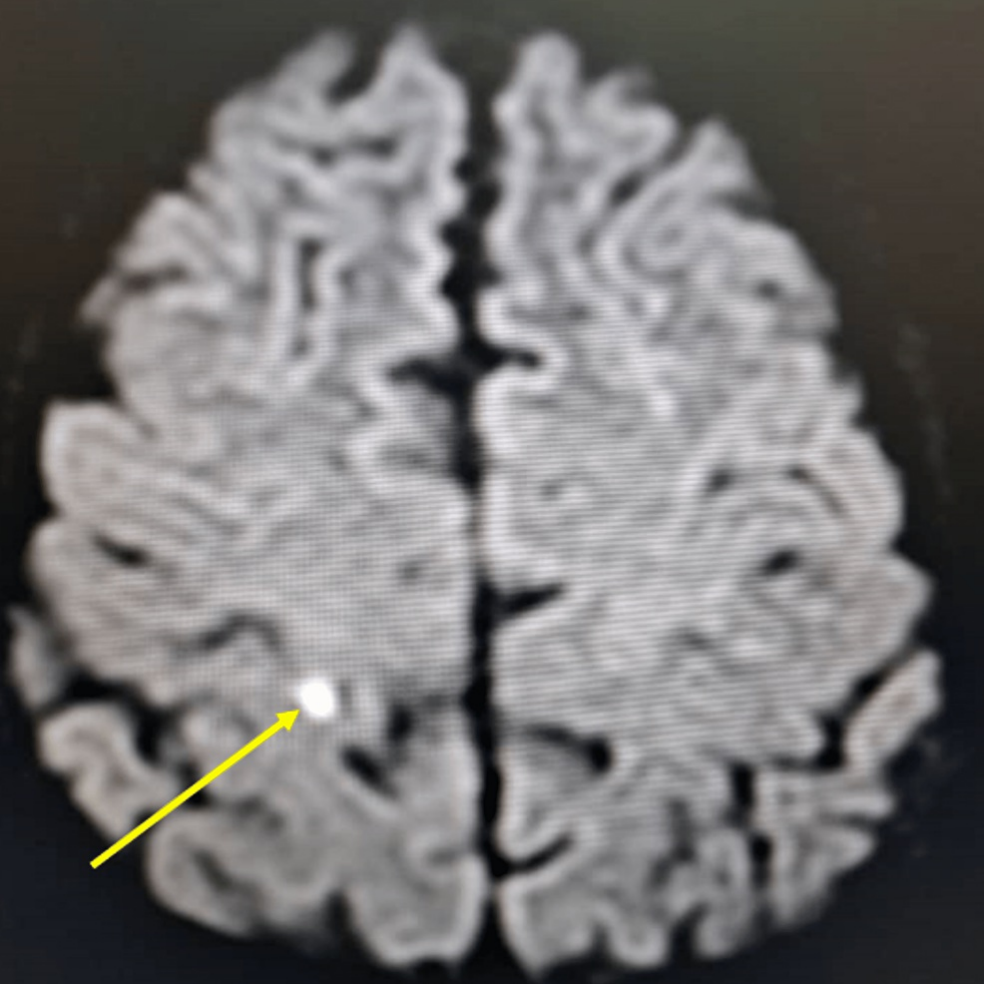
Brain MRI diffusion sequence showing small punctate signal abnormalities (hyperintensities) in the frontal and parietal lobes suggesting a lacunar ischemic stroke (embolic origin) but a WE is not to be ruled out. MRI: magnetic resonance imaging, WE: Wernicke's encephalopathy
